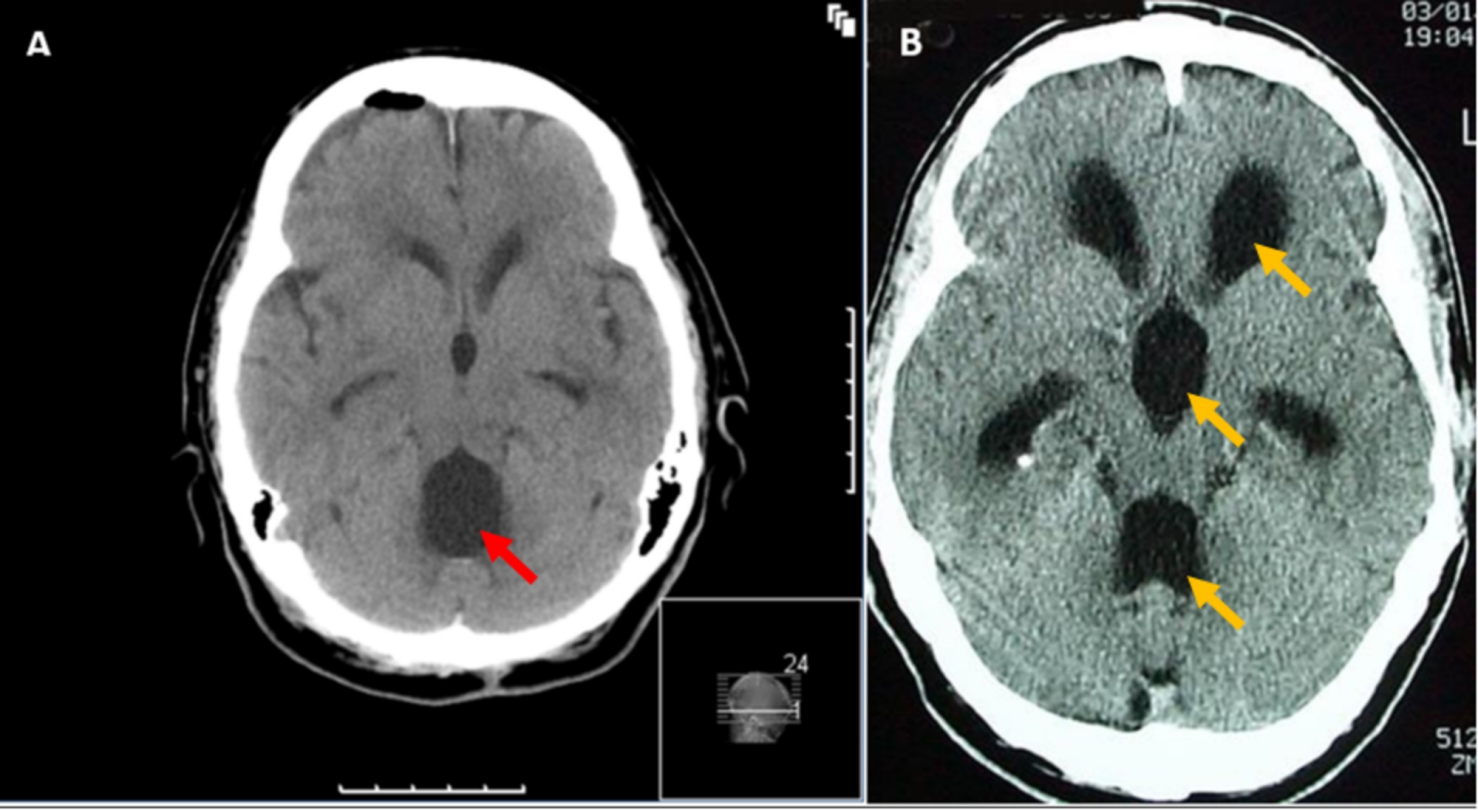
Typical CT image of DLCFV (A) compared with the proportionate enlargement of all ventricles more often seen in a communicating hydrocephalus (B). Note that in DLCFV only the fourth ventricle is disproportionately enlarged (as shown by the red arrow), compared to a universal enlargement (as shown by the yellow arrows). CT: Computed tomography; DLCFV: Disproportionately large communicating fourth ventricle.
